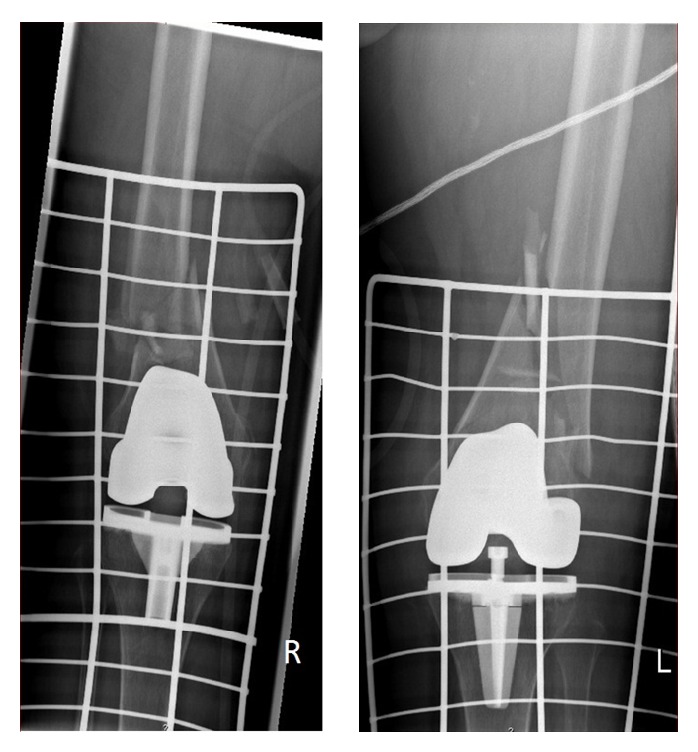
Preoperative AP X-rays showing bilateral symmetrical periprosthetic femoral fracture above knee arthroplasties.
